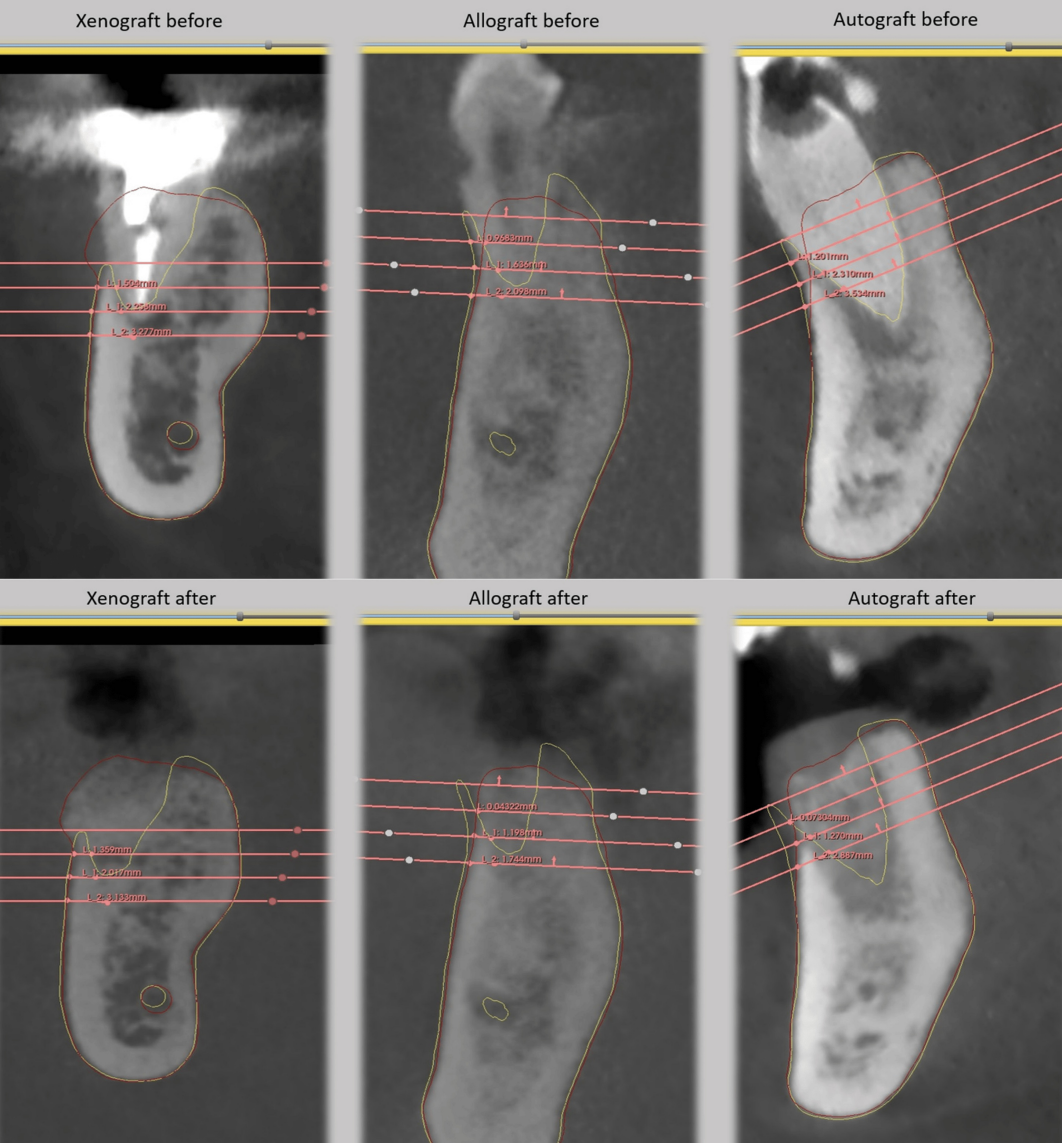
Comparison of linear CBCT measurements of buccal wall dimensions before and after grafting with xenograft, allograft, and autograft bone substitutes. CBCT, cone beam computed tomography
